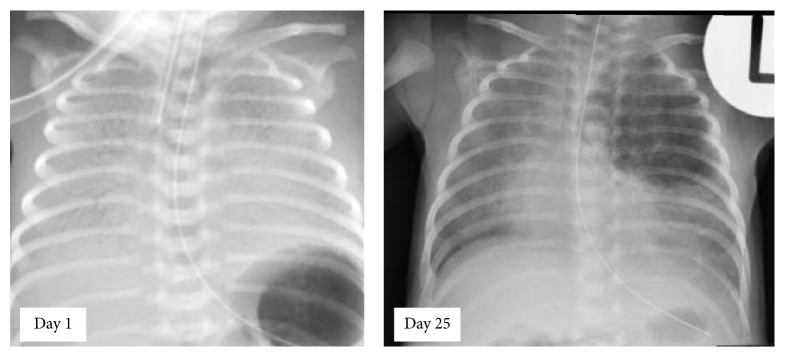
Chest radiographs of neonate showing features of hyaline membrane disease on day 1 of life and typical features of left upper lobe congenital lobar emphysema (CLE) on day 25 of life.
